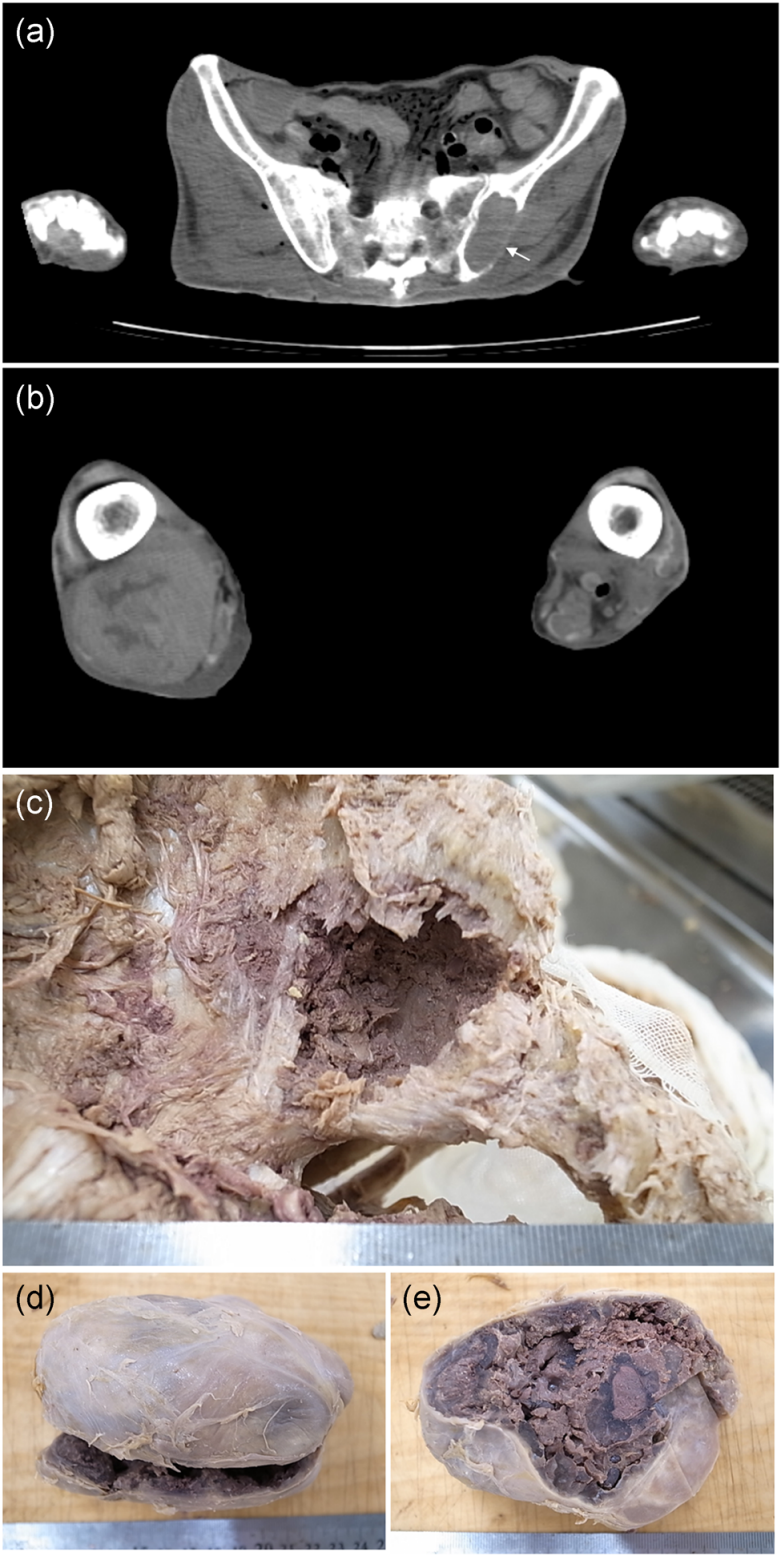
Postmortem imaging and gross appearance of anatomy Case No. 13. PMI‐CT shows nodular osteolytic change (arrow) of the left iliac bone (a) and a subcutaneous nodule of the right femoral posterior region (b). Macroscopic view of the osteolytic lesion of the left ilium (c) and removed tumor of the right femoral posterior region (d) at the time of anatomy dissection. PMI‐CT, postmortem imaging‐computed tomography.
